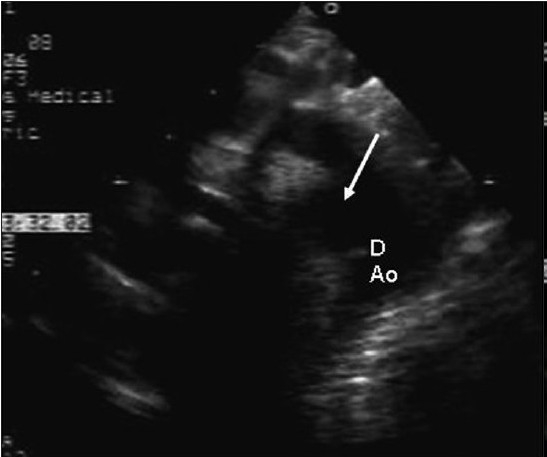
Two-dimensional echocardiography from modified suprasternal echocardiographic long axis view showing the aneurysm and the ampulla (arrow)
